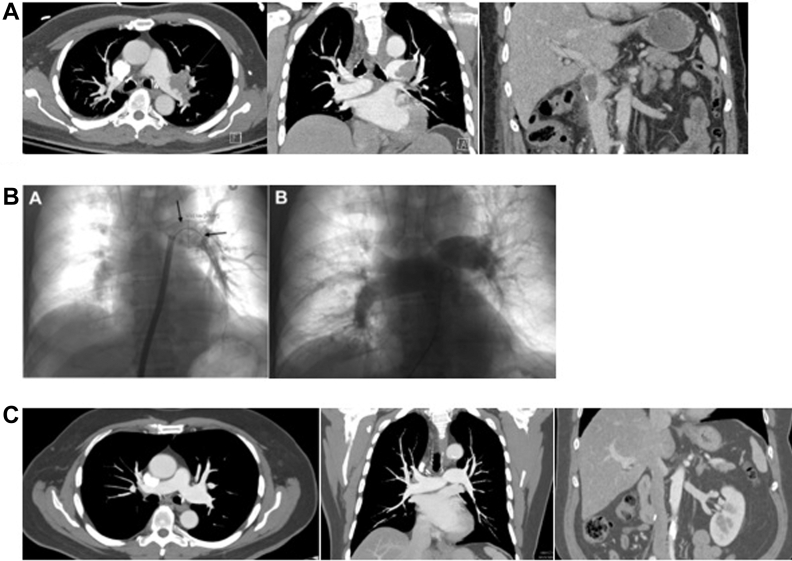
Preprocedural CT, Intraprocedural Angiography, and Postprocedural CT Images of Pulmonary Arterial Tumor Thromboembolism (A) (left to right) Axial and coronal contrast-enhanced computed tomography (CT) chest demonstrating near-occlusive left pulmonary artery thrombus. Coronal contrast-enhanced CT abdomen and pelvis demonstrating infrahepatic inferior vena cava thrombus. (B) (left to right) Intraprocedural pulmonary angiograms before and after thrombectomy demonstrating near-occlusive left pulmonary artery filling defect (arrows), which resolved with thrombectomy. (C) (left to right) Most recent follow-up axial and coronal contrast-enhanced CT chest demonstrating patent left pulmonary artery. Coronal contrast-enhanced CT abdomen and pelvis demonstrating patent infrahepatic inferior vena.
